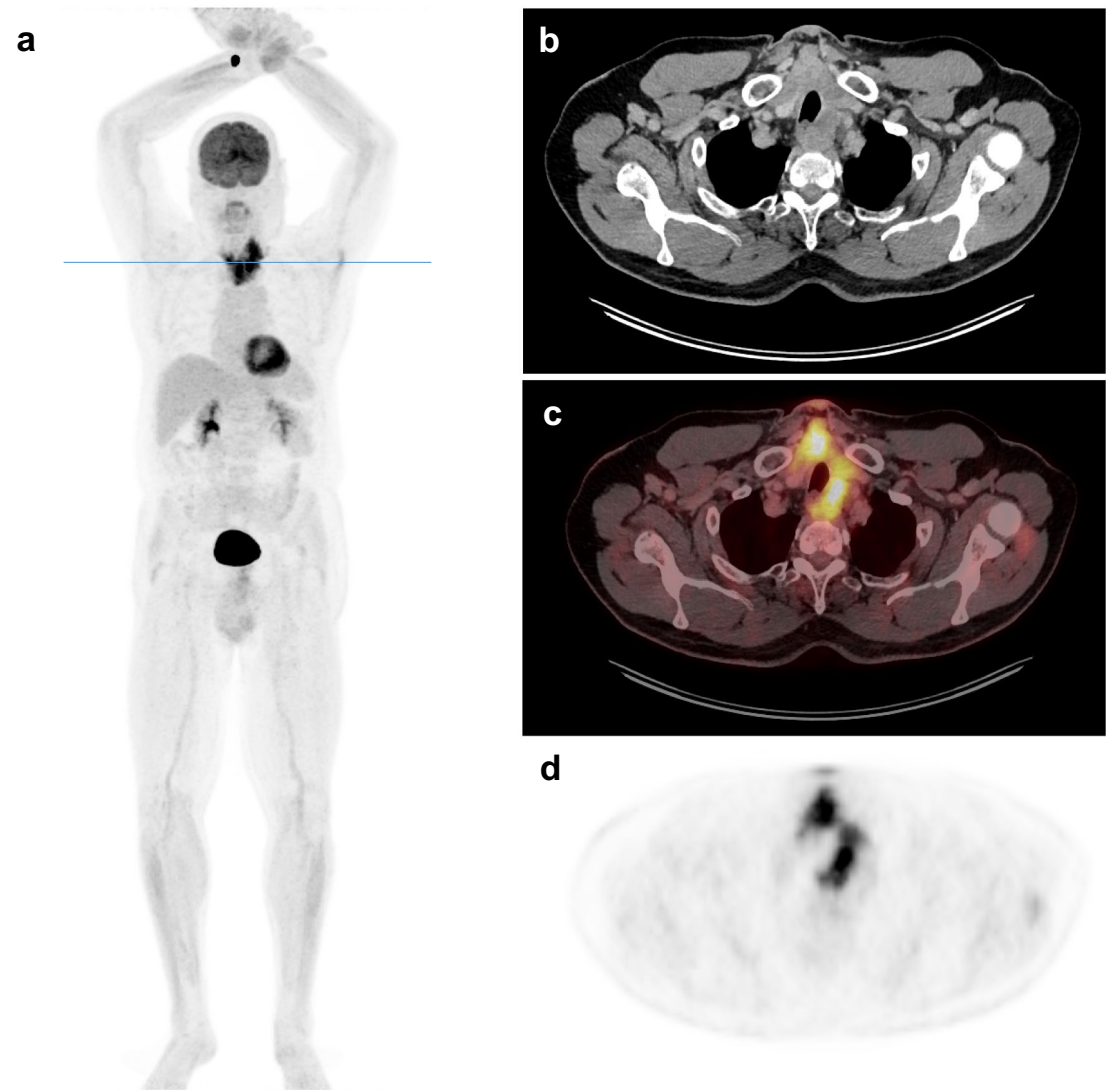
Postoperative FDG-PET/CT scan. Two weeks after surgery FDG-PET/CT scan revealed a 9.6 × 7.1 × 9 cm left cervical tumor mass expanding from the esophagus to the carotid sheath that infiltrates the trachea, the front-edge of the lower cervical vertebrae and the higher thoracic vertebrae. a Maximum intensity projections of 18F-FDG-PET. b Direct comparison of contrast-enhanced CT, c fusion imaging and d FDG-PET
